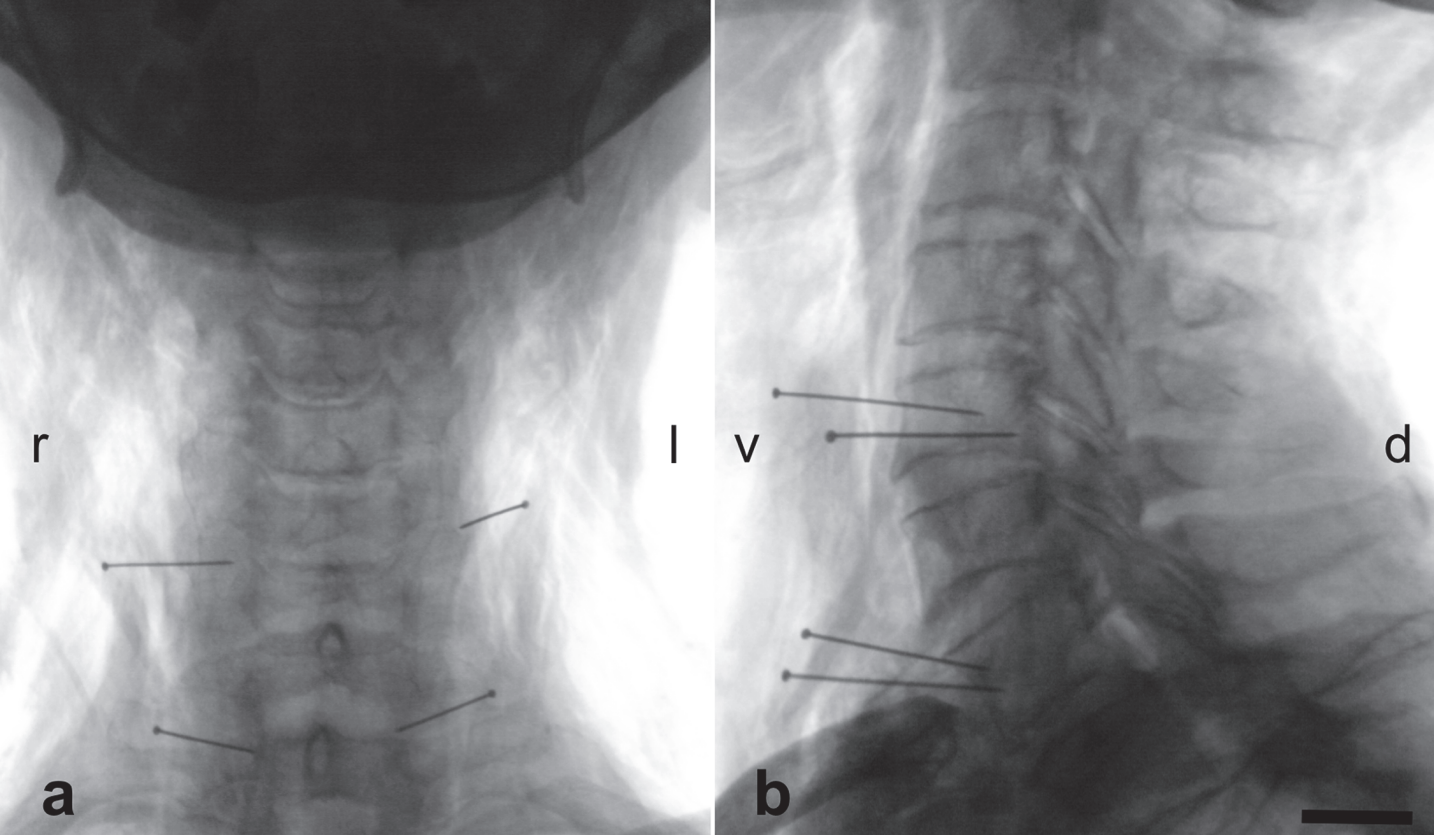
X-rays obtained from the cervical spine of a 69 year-old male in the anterior-posterior (3a) and in the lateral projection (3b). Metal needles indicate the most cranial and caudal part of the vagus nerve that could be visualized with the surgical approach to the carotid triangle. d = dorsal, l = left, r = right, v = ventral; scale bar = 10 mm.
